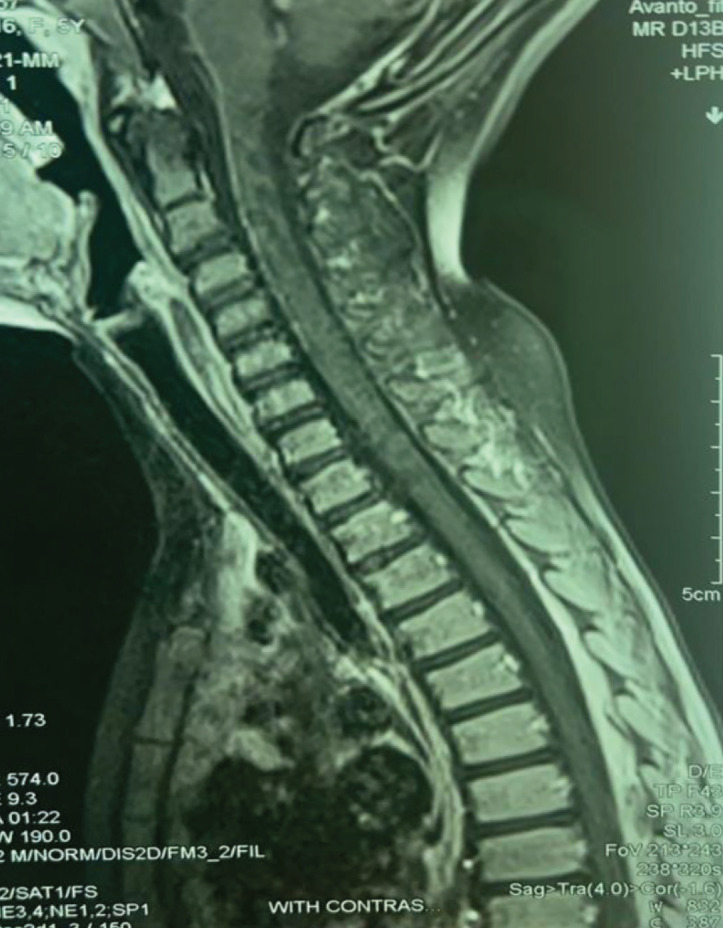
Spinal MRI upon addmision
